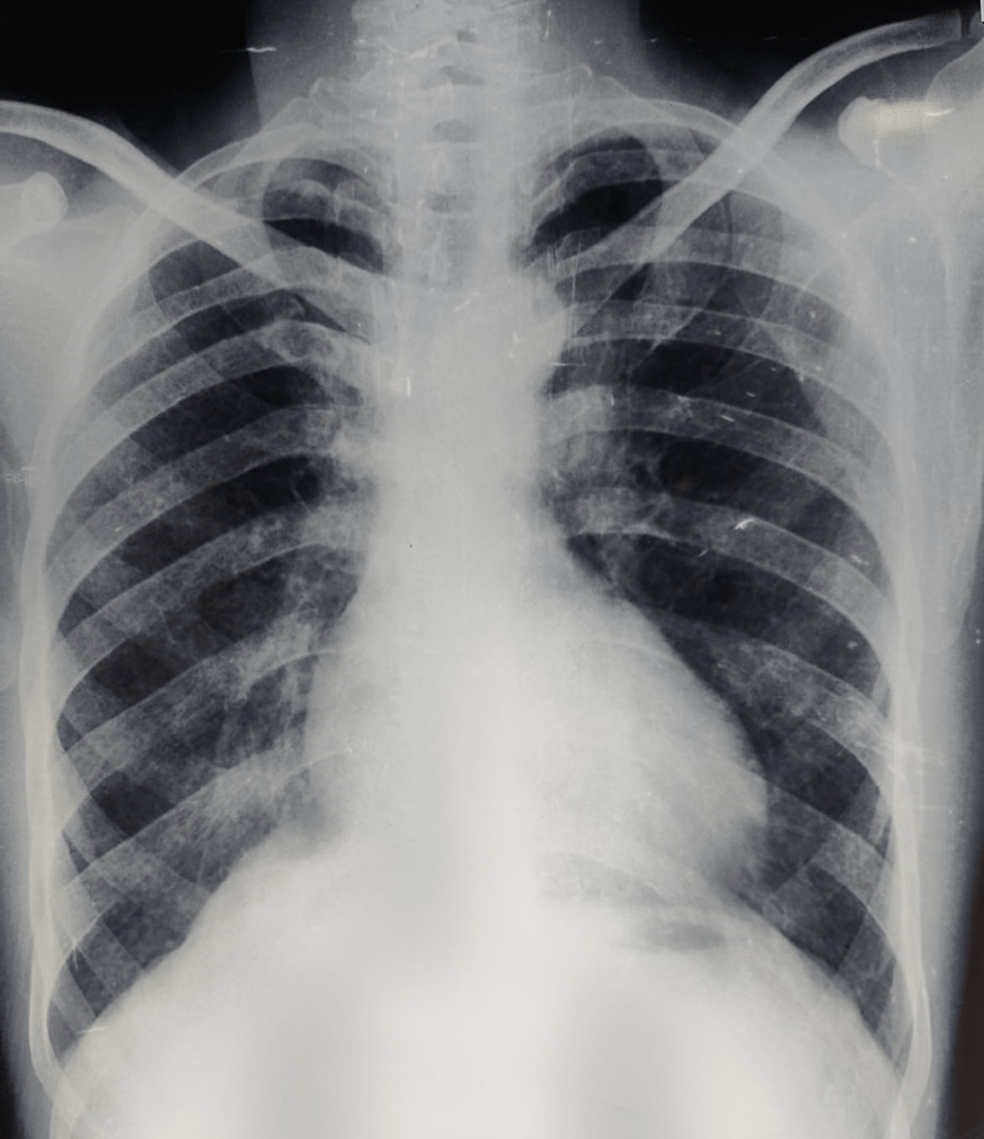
Chest X-ray PA view showing mild cardiomegaly with right ventricular type apex. PA = posteroanterior
